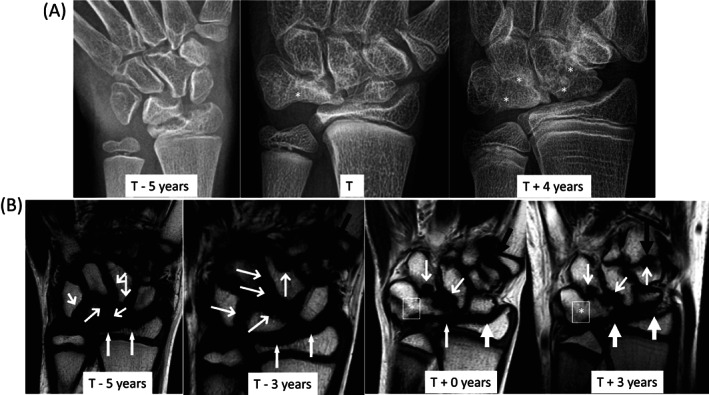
Images of left wrist. (*A*). Frontal radiographs of left wrist demonstrate multiple erosions in the distal radius, carpus, and proximal metacarpals with progressive carpal fusion (*) and loss of joint space. In posttreatment, overgrown distal radius and ulna epiphyses and multiple growth recovery lines are visible. (*B*) T1 signal‐weighted MR images of left wrist demonstrate to advantage numerous erosions in the radius (thick black arrows), ulna, carpals (thin black arrows), and metacarpal bases (white arrow). By 2017, the lunate and triquetrum have fused. By 2020, very little carpal joint space remains. MR = magnetic resonance.
